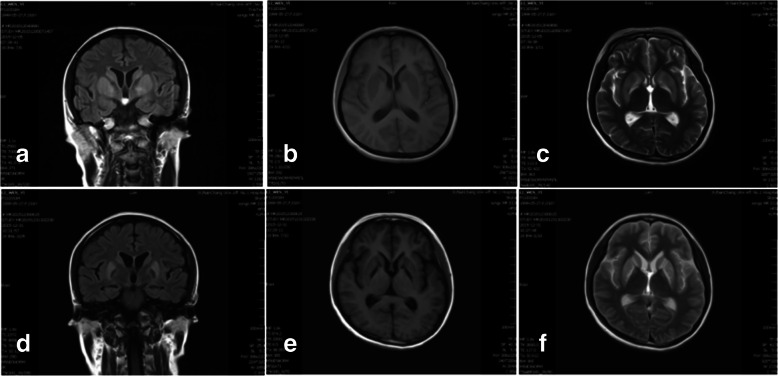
Postoperative day 20 and 49 MR images. a, b, c: coronal FLAIR MR image (a) taken on postoperative day 20 showed symmetrical bilateral hyperintense lesions in the lenticular nucleus. Axial T2-weighted MR image (c) showed markedly hyperintense signal in the same area. d, e, f: coronal FLAIR MR image (d) taked on postoperative day 49 showed the lesions with increased intensity and axial T1-weighted MR image showed hypointense abnormality in the basal ganglia area. Axial T2-weighted MR images (f) showed the lesions even worse, hyperintense lesions developed in bilateral caudate nucleus and lenticular nucleus
